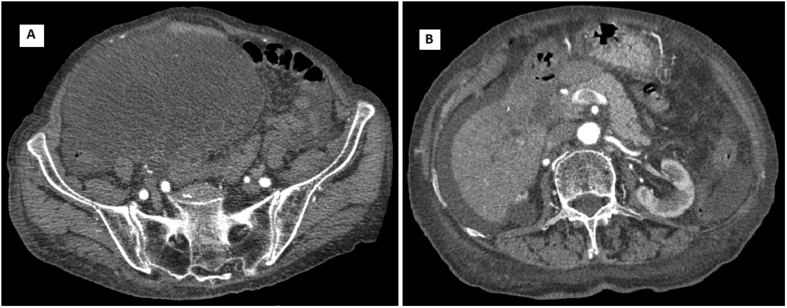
Abdominal CT scan with axial views revealed a voluminous mass in the right iliac fossa (A), the presence of ascites and a thick-walled gallbladder adherent to the duodenum (B).
